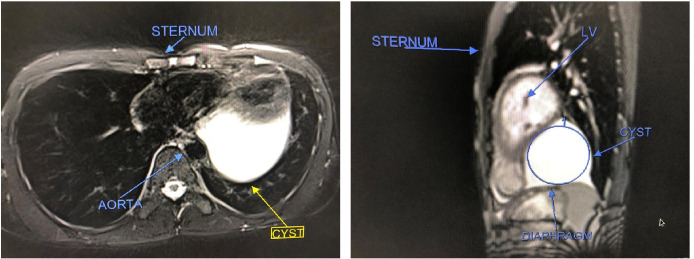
MRI in transverse section and sagittal view showing a well-defined cystic mass in the posterior and lateral portion abutting the left cardiac border.
